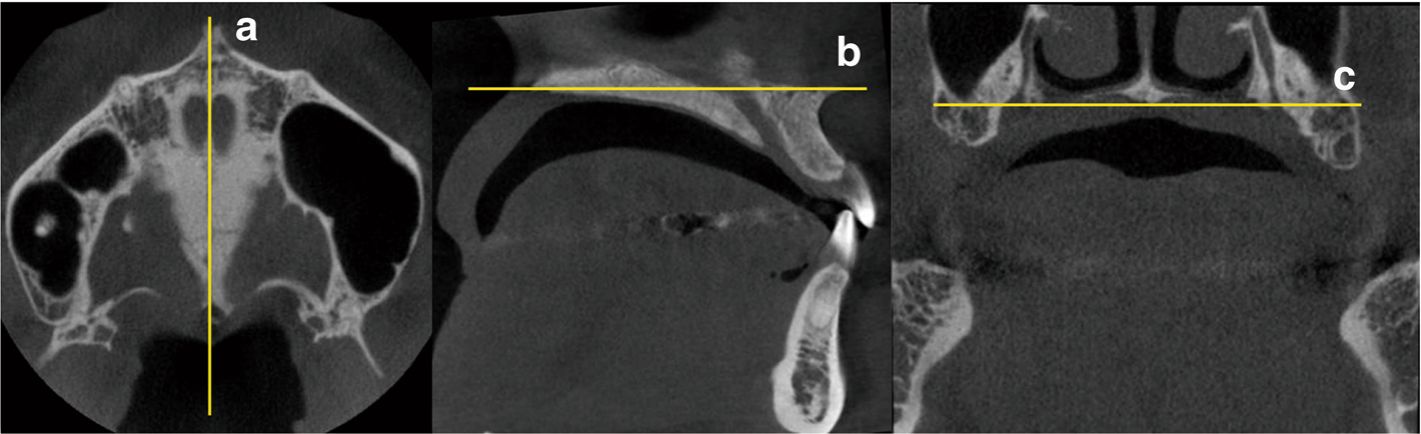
Landmarks*, *reference lines*, and *planes* drawn on cone-beam computed tomography images. a Sagittal plane – passing through the midpalatal suture, perpendicular to the horizontal and coronal planes. b Horizontal plane – the palatal plane. c Coronal plane – passing through the *right* and *left* greater palatine foramina. Abbreviations: *R* right, *L* left, *P* posterior, *A* anterior
